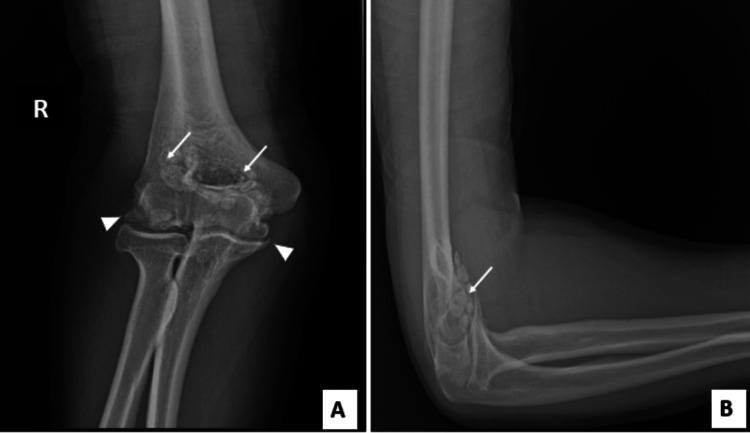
X-rays of the right elbow (A) anteroposterior view (B) lateral view Revealed multiple intra-articular loose bodies (arrow), pinching of the joint space, subchondral sclerosis, and osteophytes (arrowhead).
